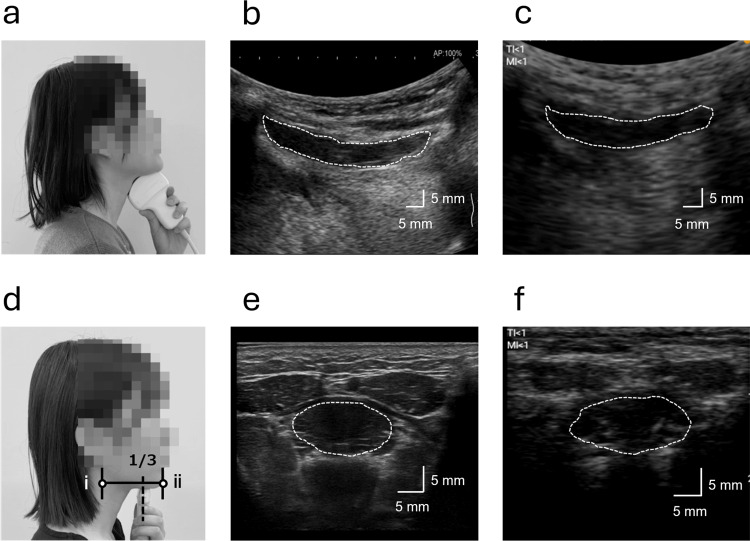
Ultrasound measurement and B-mode imaging of the geniohyoid muscle (GM) The regions enclosed by white dotted lines in the B-mode images represent the GM muscle belly. (a) Ultrasound measurement in the midsagittal plane. The longitudinal cross-section of the GM was recorded. (b) B-mode image obtained using the ultrasound imaging system (UIS) in the midsagittal plane. (c) B-mode image obtained using the handheld ultrasound device (HHUD) in the midsagittal plane. (d) Ultrasound measurement in the coronal plane. The measurement site was located approximately one-third of the distance from the anterior (ⅰ) to the posterior (ⅱ) margin of the mandible. (e) B-mode image obtained using the UIS in the coronal plane. (f) B-mode image obtained using the HHUD in the coronal plane.
